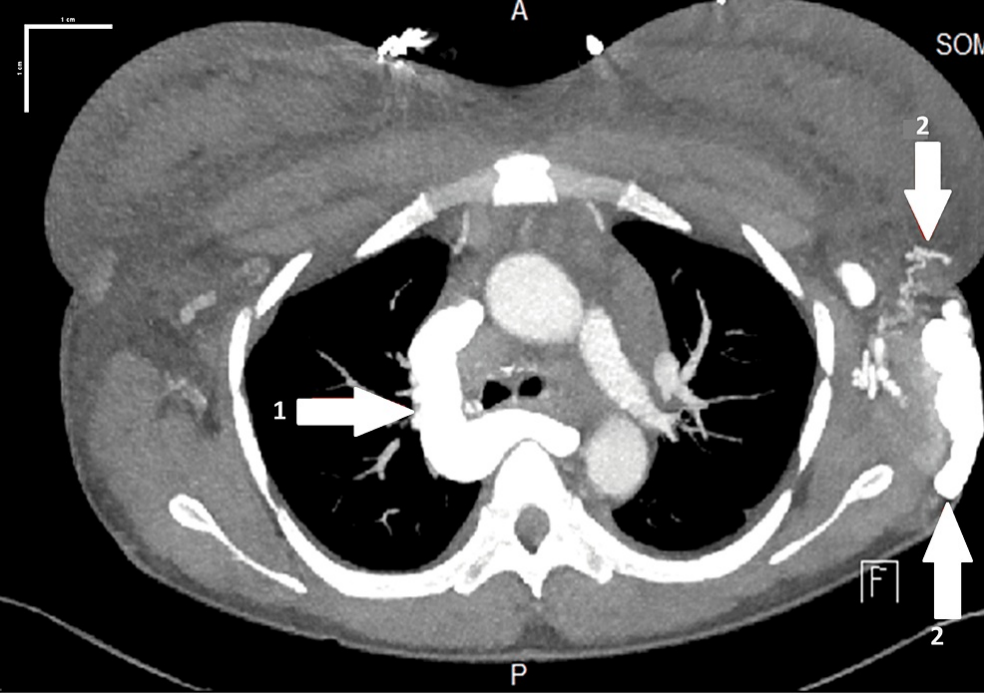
Axial section computed tomography angiography (scale bar, 1 cm) shows, via label 1: a hypertrophied azygos vein, and via label 2: hypertrophied venous collateral vessels along the left chest wall.
